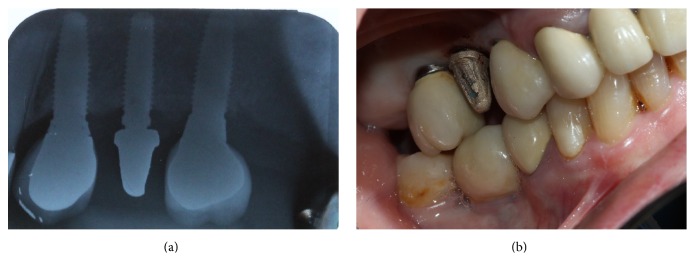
Sealing and adaptation were made: (a) radiographic control and (b) disclosing wax verifications.
